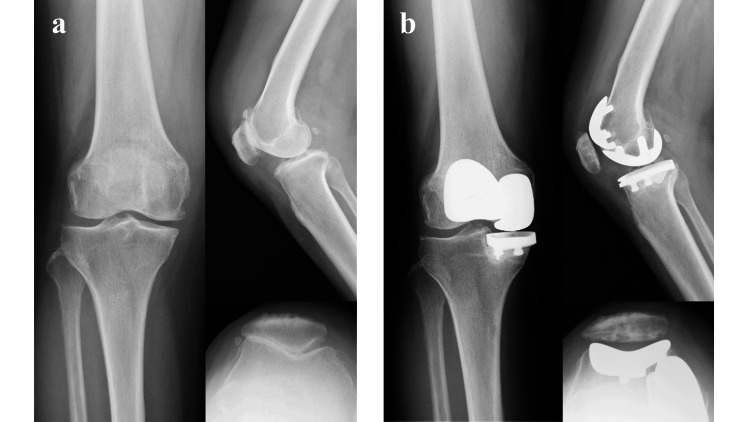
Preoperative (a) and postoperative (b) radiographs of a bicompartmental knee arthroplasty (BKA).
